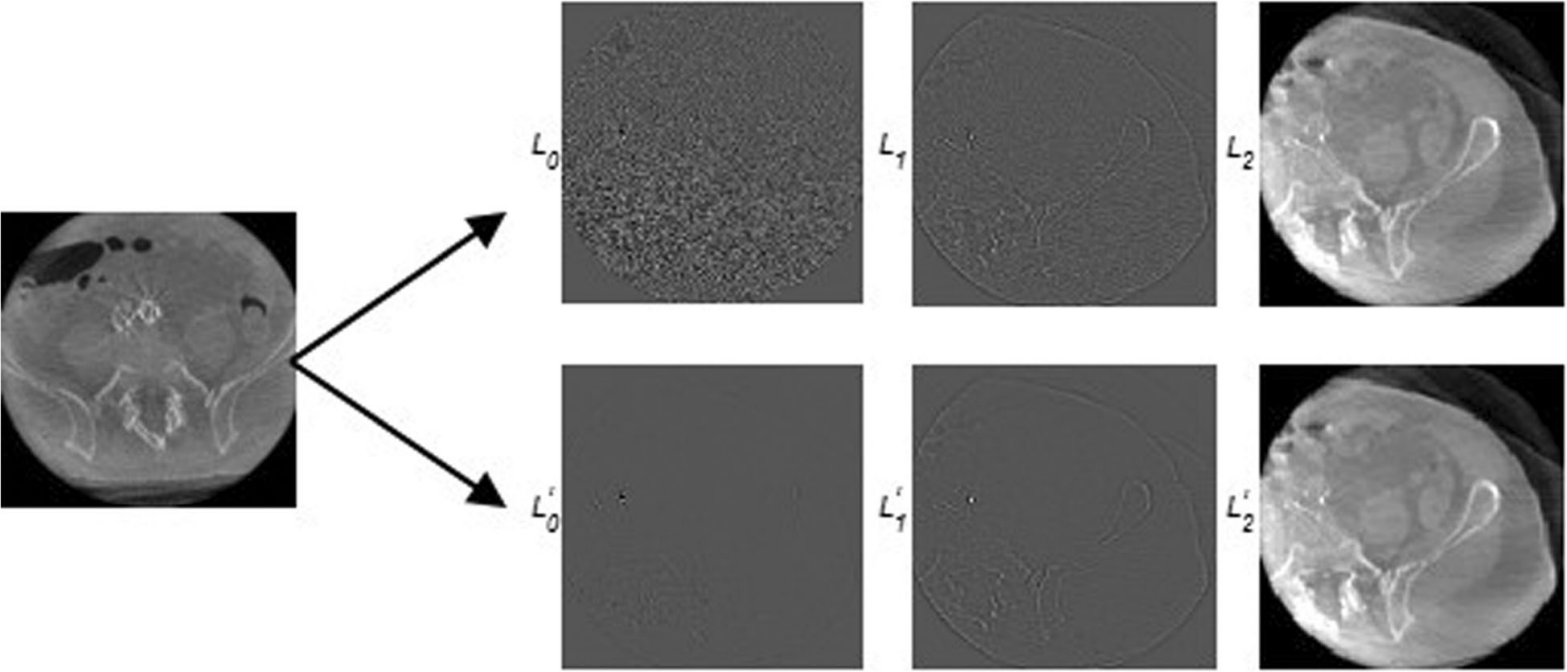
Noise and artifacts suppression in 3 levels of Laplacian pyramid for the degraded CBCT image. Left column shows the original image; right top row shows its 3 levels of Laplacian pyramid; right bottom row shows the restored Laplacian pyramid levels by MND filter
